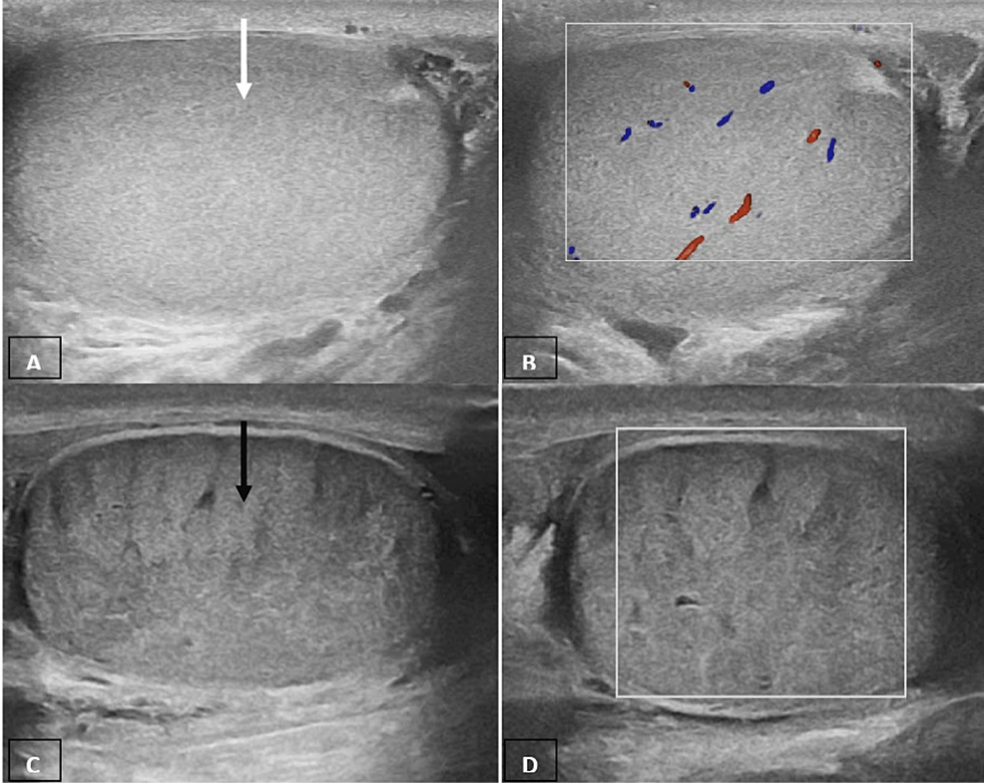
Acute torsion A, B Grey-scale and colour Doppler images of a normal right testis (solid white arrow); C, D grey-scale and colour Doppler images of the left testis (solid black arrow) that shows a heterogeneous echotexture and no vascularity.
